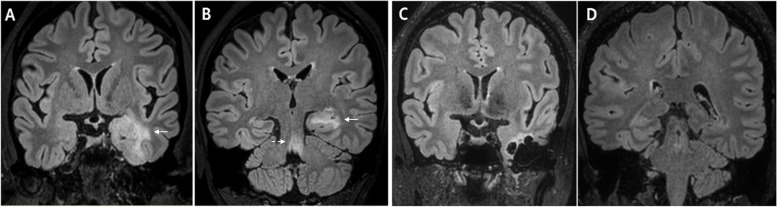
A, B FLAIR images showing infiltrative hyperintense lesions in the left medial temporal lobe (arrows) and the left dorsal pons (dashed arrow). C, D Follow-up MRI obtained 2 years showing a marked decrease in the extent of hyperintense lesions with cystic changes related to the biopsy
